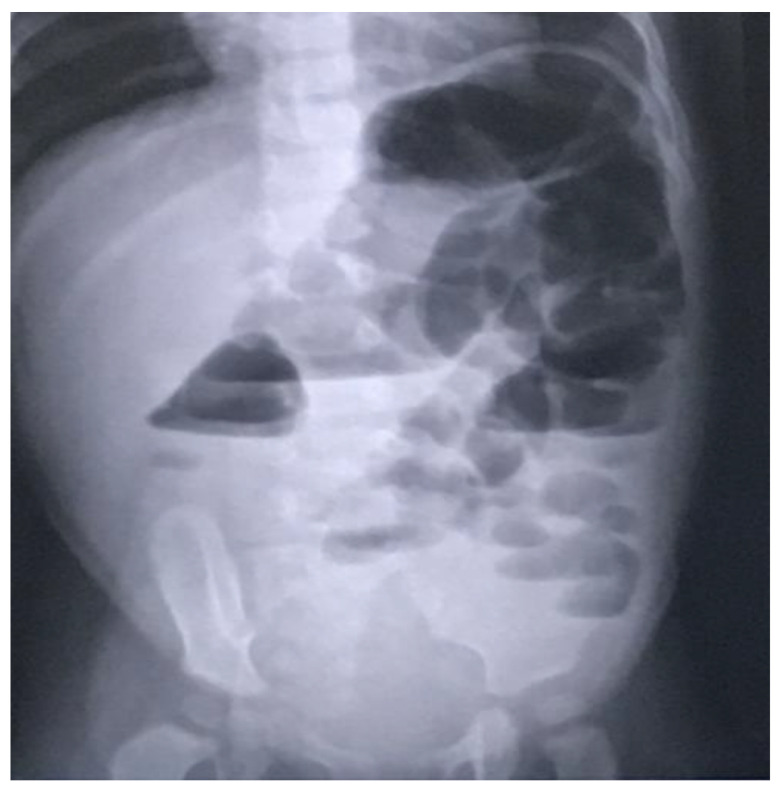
Radiographic indications of bowel distension with discernible air-fluid levels and the nonexistence of any lesion causing luminal occlusion.
